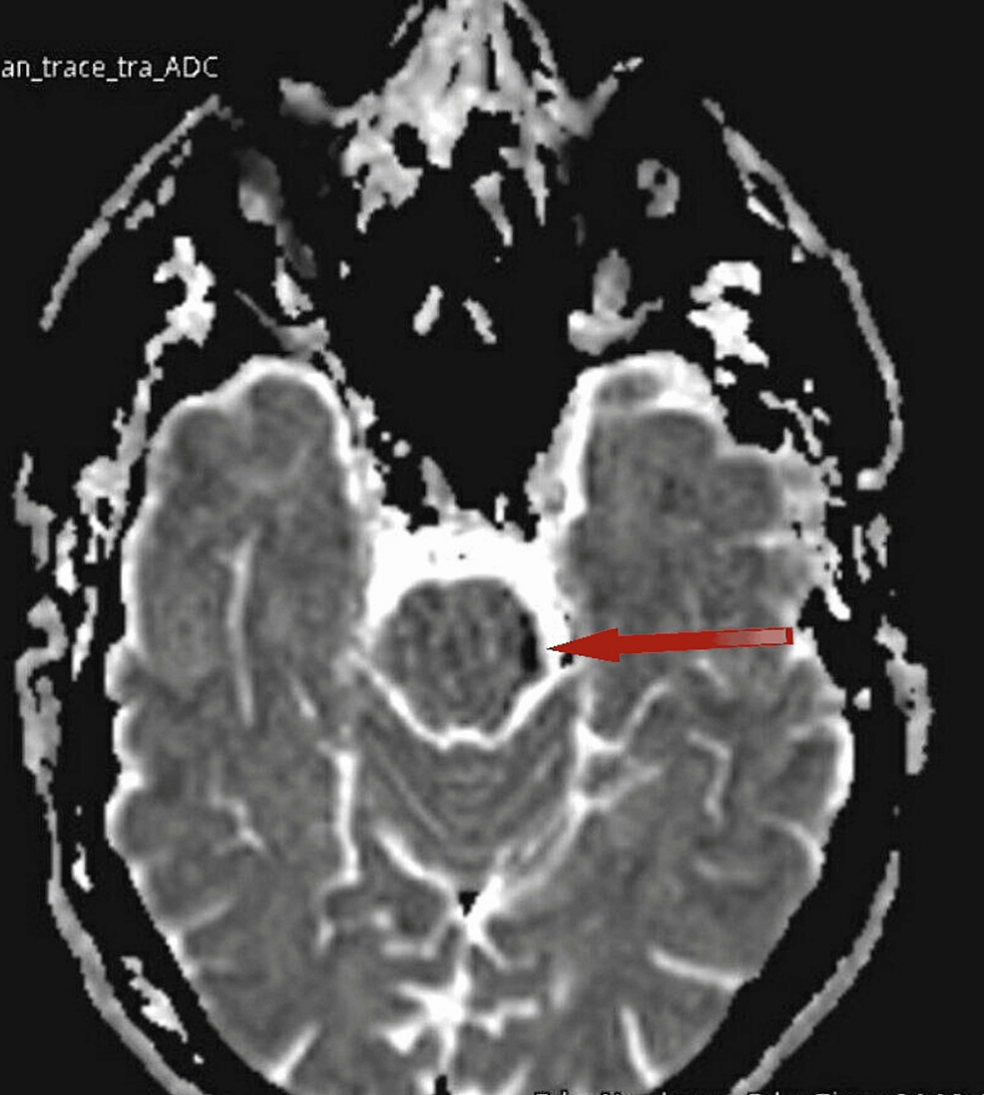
An ADC MRI image demonstrating low signal indicating ischemic infarct (red arrow) ADC: apparent diffusion coefficient; MRI: magnetic resonance imaging
